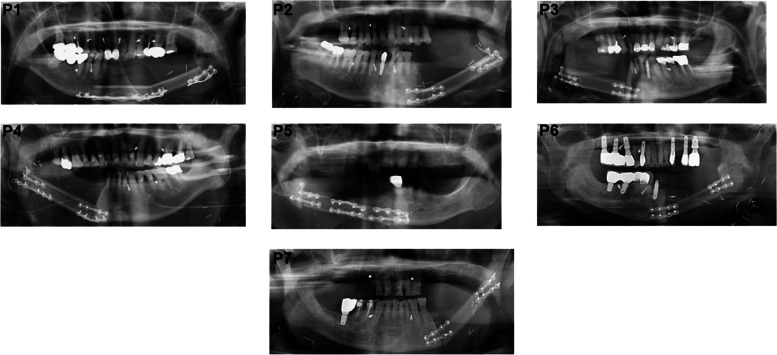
Postoperative orthopantomogram X-rays of the patients
